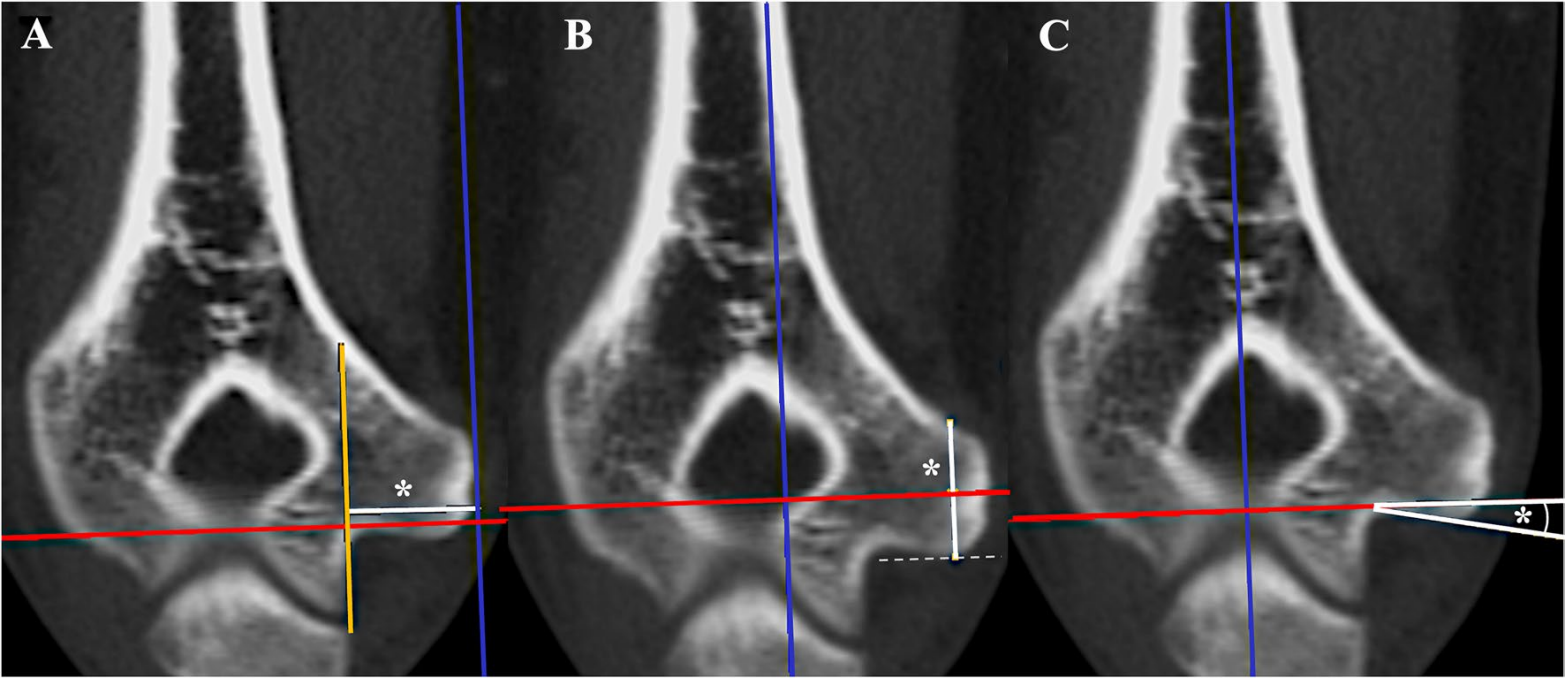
Measurements in the coronal plane. Off- set of the medial epicondyle (ME) (a), height of the ME (b), and the coronal angulation of the inferior ME (c) are outlined. *: Point of measurement; blue line: sagittal plane; red line: axial plane. Orange line in (a) is drawn parallel to the sagittal plane and represents the medial boundary of the trochlea, while the sagittal plane is adjusted on the most medial point of the ME. The dotted line in b indicates the most distal point on the ME. All pictures are taken in the same coronal plane of the same CT scan
